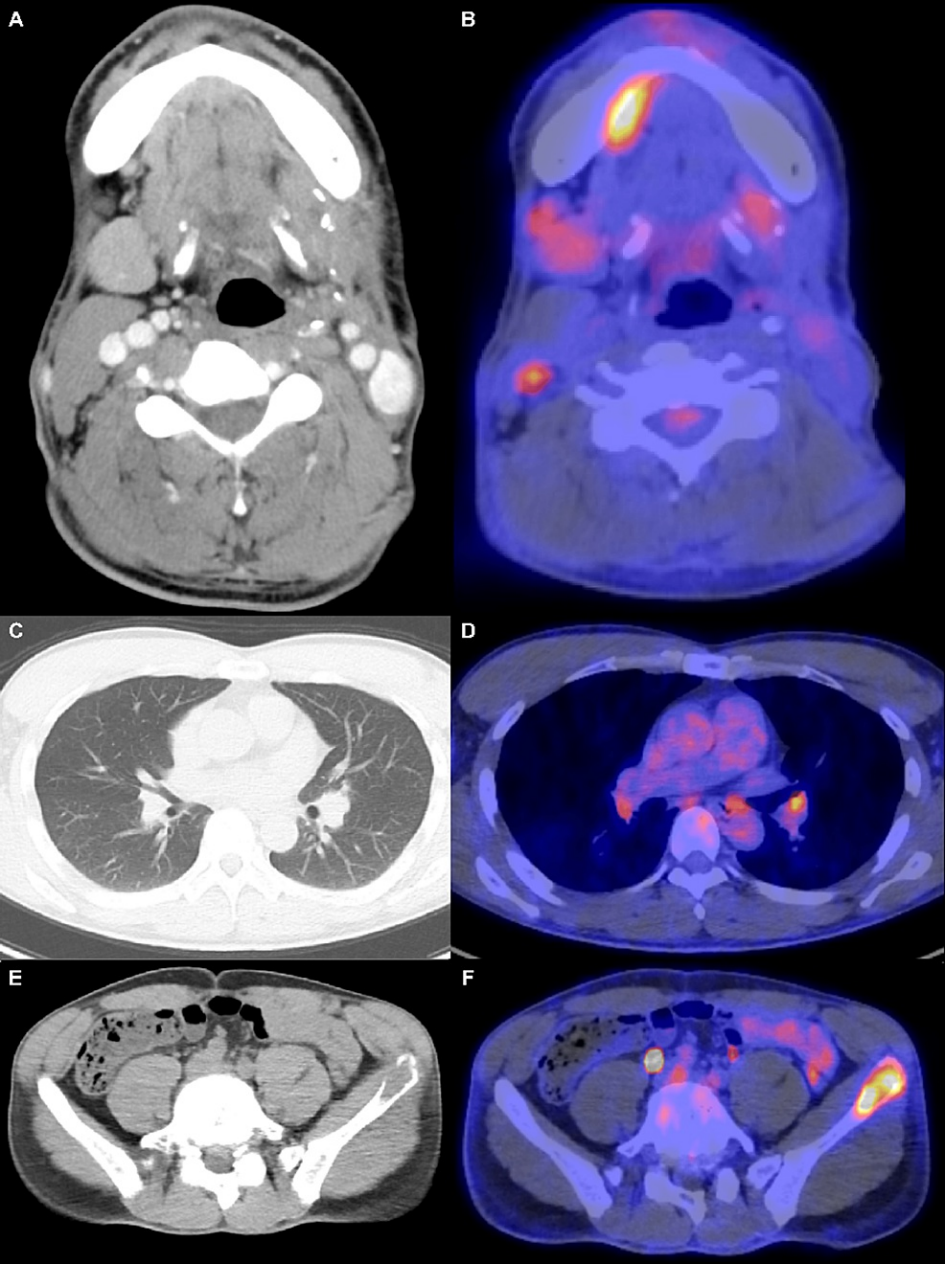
Radiological findings of the tumor in Case 1 after nivolumab administration. Findings after 10 courses of nivolumab administration. Contrast-enhanced CT and FDG-PET revealed enlargement of each target lesion, indicating progressive disease (A-F).
